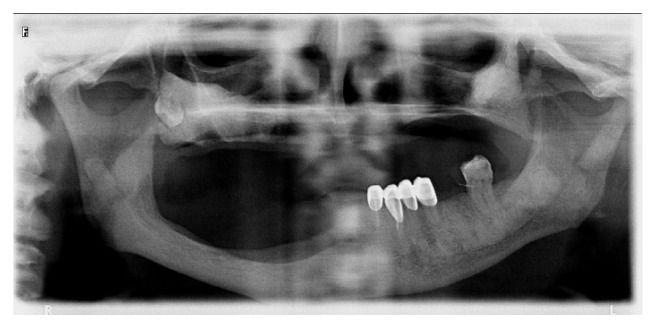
Panoramic radiography view of patient before rehabilitation with prostheses in 2014.
